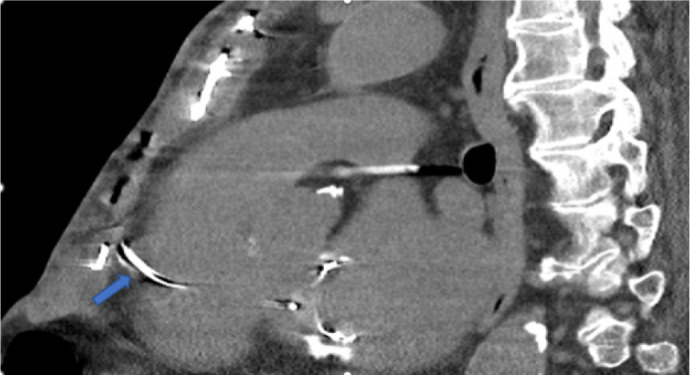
Non-contrast chest computed tomography: sagittal view, demonstrates
